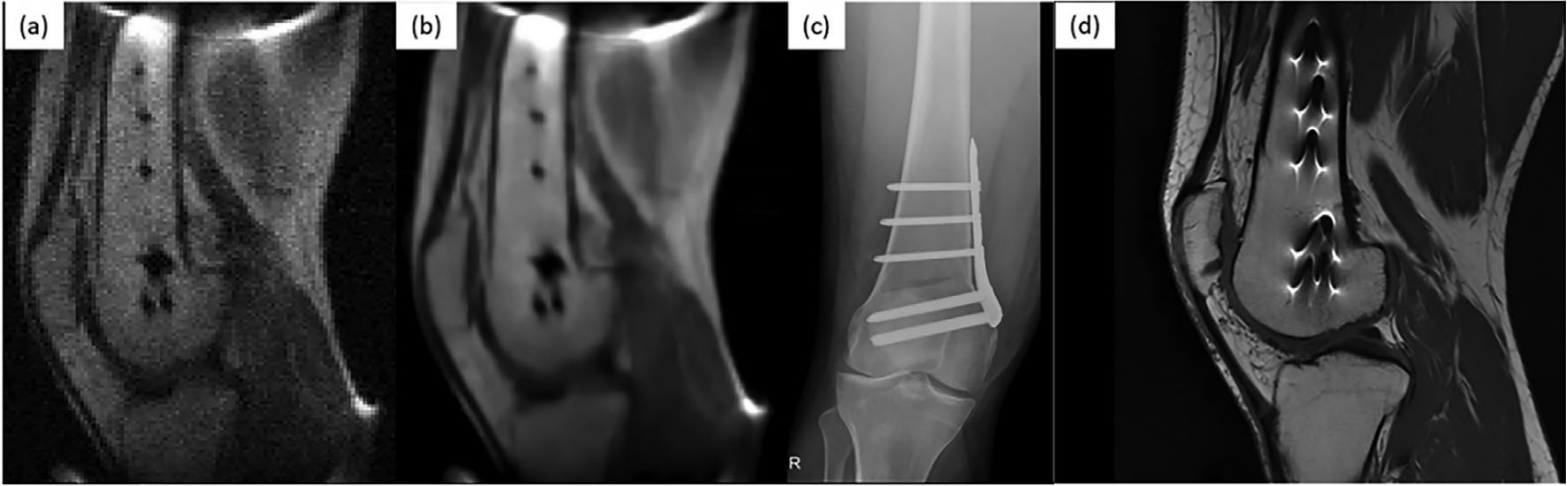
Image modified from Guallart‐Naval et al.^84^ Image of fixation metallic implant attached to the femur, consisting of a plate and seven screws: (A) (9 mm slice from $T_{1}$‐weighted 3D‐RARE acquisition with in‐plane resolution of 1.3 × 2 mm^2^, 12 min scan time, 8 years after femoral shaft osteotomy); (B) same, but BM4D‐filtered and rescaled by ×2 to increase the number of pixels; (C) lateral X‐ray computed radiography (2 weeks after surgery); (D) sagittal view of the same knee, acquired with a Siemens Skyra 3 T system ($T_{1}$‐weighted 2D‐RARE acquisition with slice thickness 3.9 mm and pixel resolution 0.26 × 0.26 mm^2^, 1 year after surgery).
